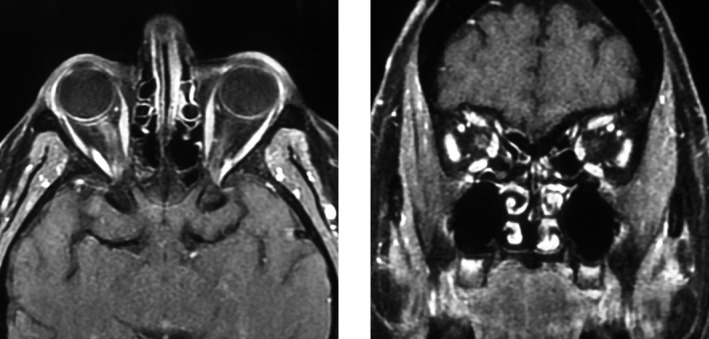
MRI orbits, axial and coronal sections, show enhancement of right optic nerve sheath
